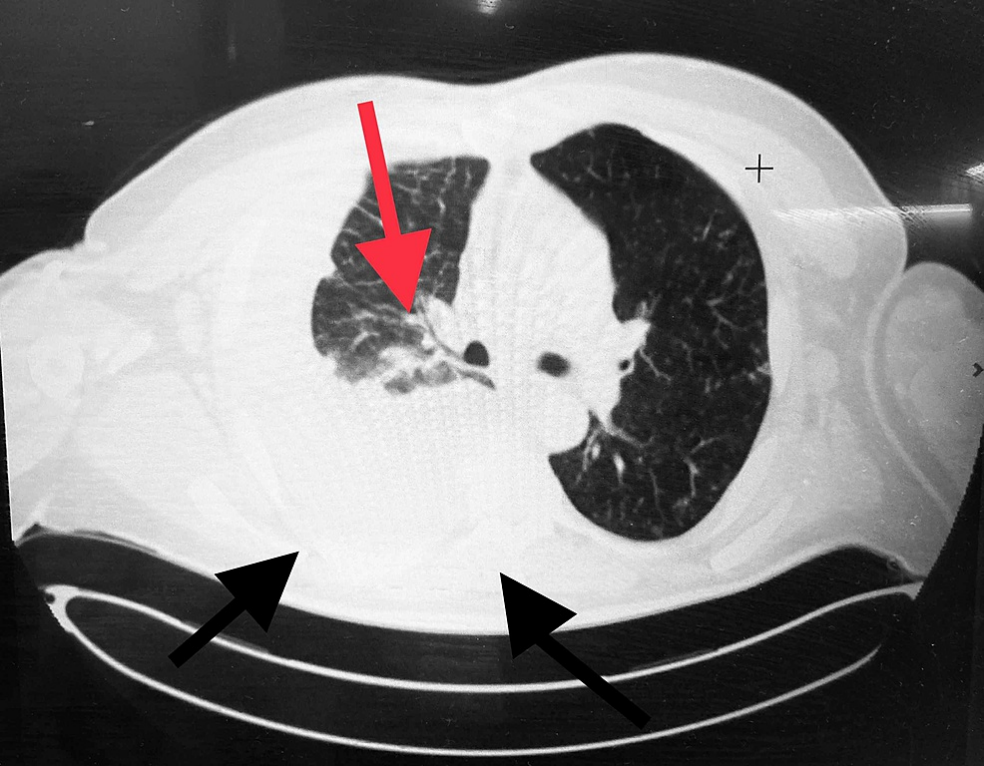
Chest CT with intravenous contrast. Two black arrows showing large loculated right pleural effusion, small cardiac effusion and mediastinal adenopathy (red arrow).
